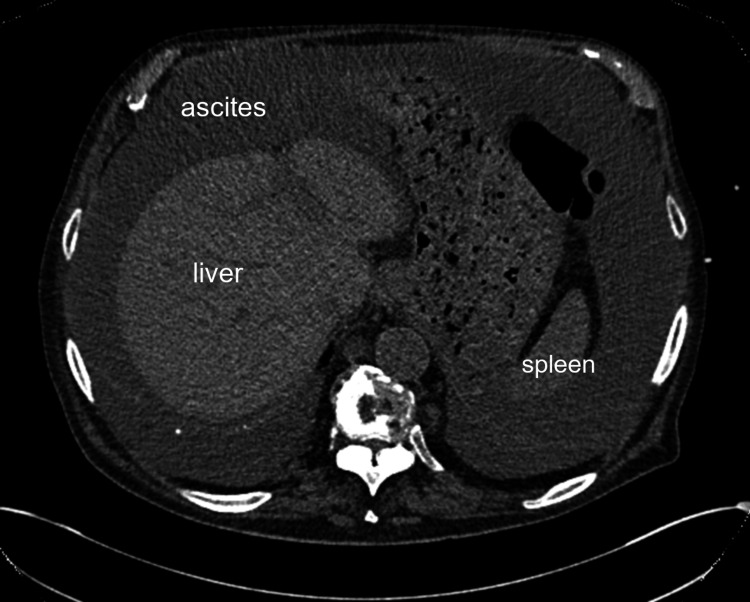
Non-contrast thoracoabdominal-pelvic CT scan (axial view) showing abundant ascites
